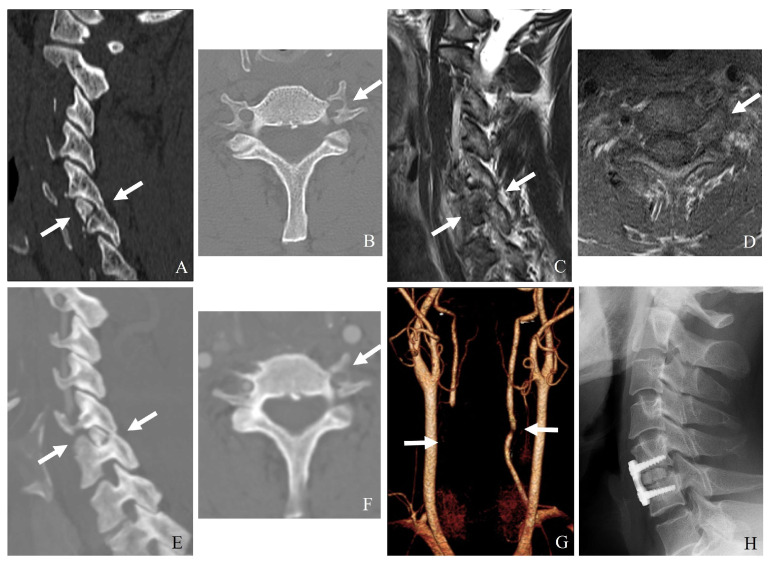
Computed tomography (A,B) and magnetic resonance imaging (C,D) show C5–6 unilateral facet dislocation and a left transforaminal fracture fragment (white arrows). Neck computed tomography angiography (E–H) indicates left vertebral artery injury due to the transforaminal fracture fragment and mild right vertebral artery occlusion (white arrows). The patient underwent anterior cervical discectomy and fusion of C5–C6 (H).
